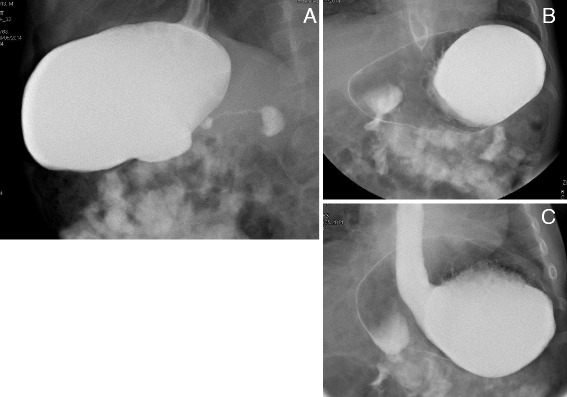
The radiographic study of the transit gastroesophageal barium meal with a projection. Lateral (a) and anteroposterior (b and c) views show marked reduction in the caliber of the pyloric region (a), the arrangement of the greater and lesser curvature (b) and reflux of contrast up to the middle third of the esophagus
